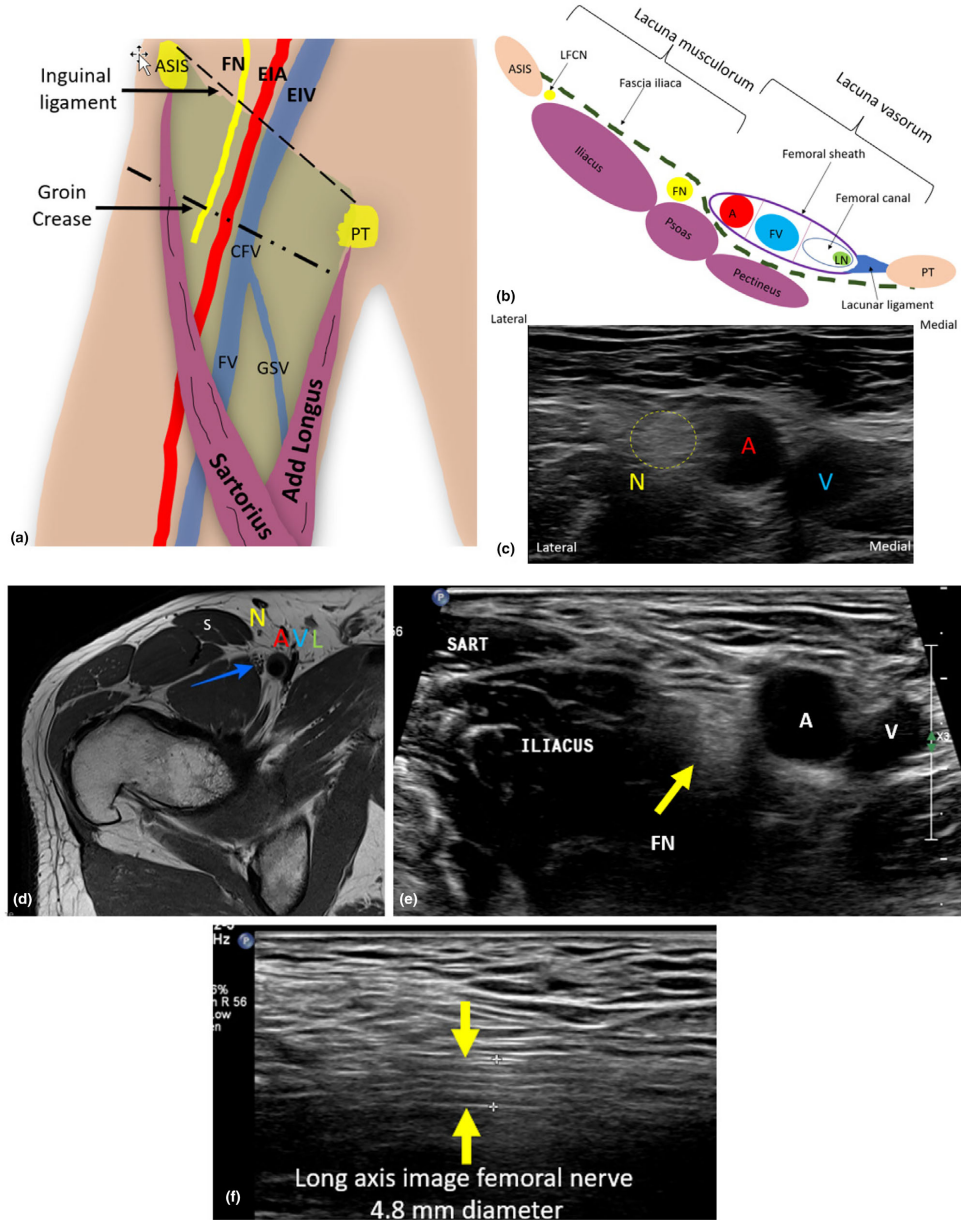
Relative position of the right femoral nerve (FN). (a) The inguinal ligament extends between the anterior superior iliac spine (ASIS) and pubic tubercle (PT). At this level, the FN sits lateral to the external iliac artery (EIA) and external iliac vein (EIV). The inguinal ligament forms the superior border of the femoral triangle (green), which is outlined by the sartorius and adductor longus muscles. (b) Cross‐sectional view of the groin, demonstrating the femoral sheath and femoral canal medially, which contain a potential space, and the lymph node of Cloquet (LN). The FN and lateral femoral cutaneous nerve (LFCN) both lie deep to the fascia iliaca. (c) A sonographic short‐axis image of the right groin, demonstrating the lateral‐to‐medial relationship of the femoral nerve (N) outlined by yellow dotted line, artery (A) and vein (V). (d) Axial MRI demonstrating the relationship between the FN (blue arrow) and the sartorius muscle (S) lateral to it. (e) Short‐axis image of the femoral nerve (yellow arrow) just superior to the groin crease, demonstrating the sartorius muscle lateral to the FN. (e, f) Long‐axis sonographic image of the FN (outlined between yellow arrows). A, artery; CFV, common femoral vein; FV, femoral vein; GSV, great saphenous vein; L, region of lymphatic channels; N, femoral nerve; SART; sartorius muscle; V, vein.
